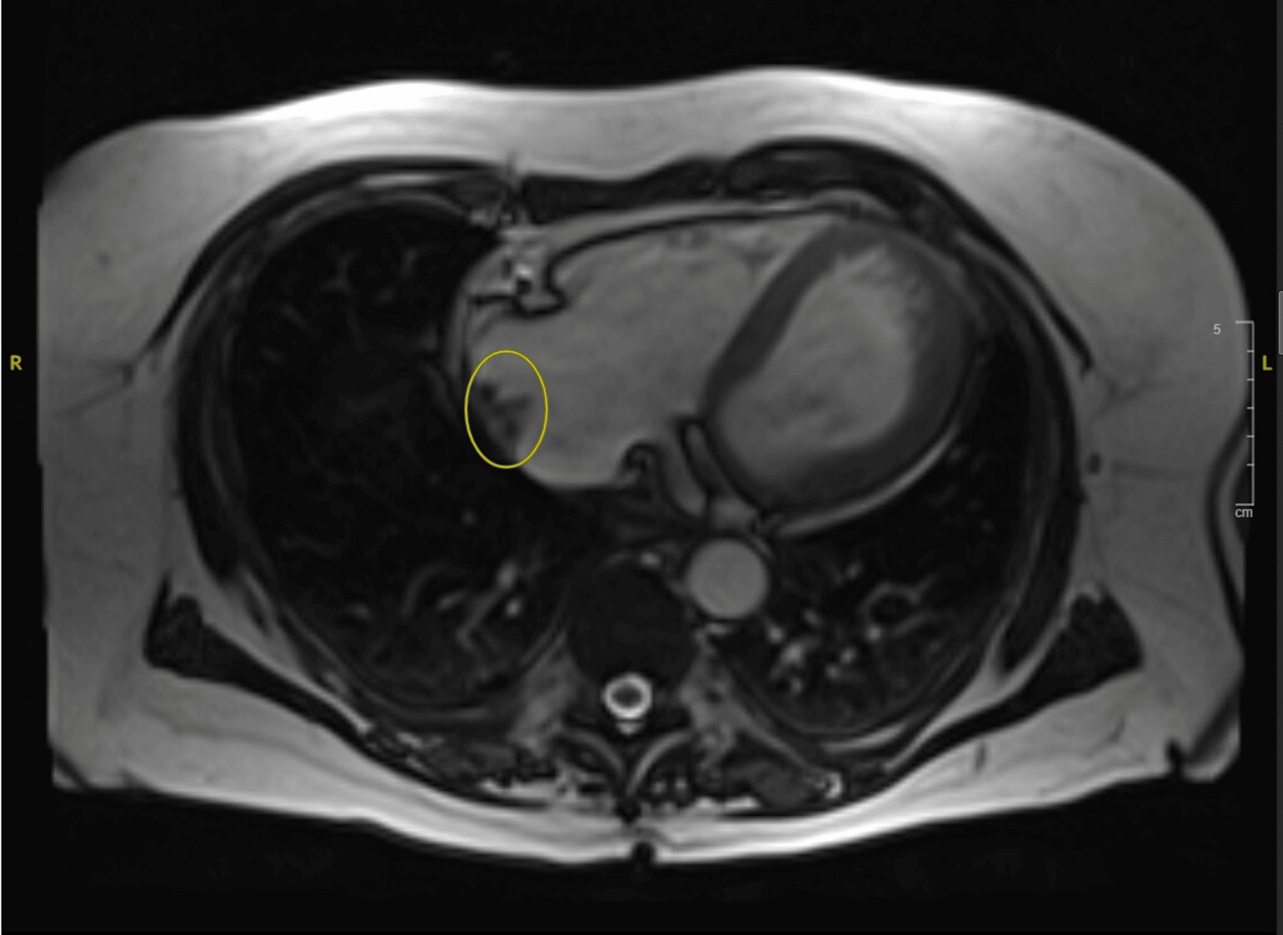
Cardiac magnetic resonance imaging (CMRI), T2-weighted axial view, showing a right atrial mass on the lateral wall measuring 1.9 × 1.2 cm. The right atrium is mildly enlarged. First-pass contrast imaging was not obtained due to technical issues, and no obvious delayed enhancement was seen in the lesion.
